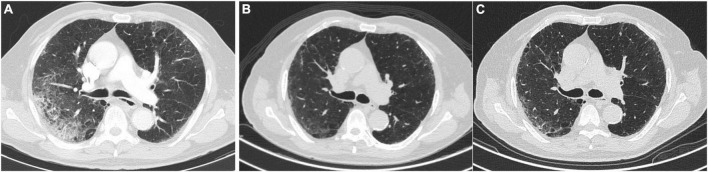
Changes in the CT scan in a patient with COVID pneumonia. (A) Upon admission. (B) At 3 months of follow-up. (C) At six-month follow-up.
